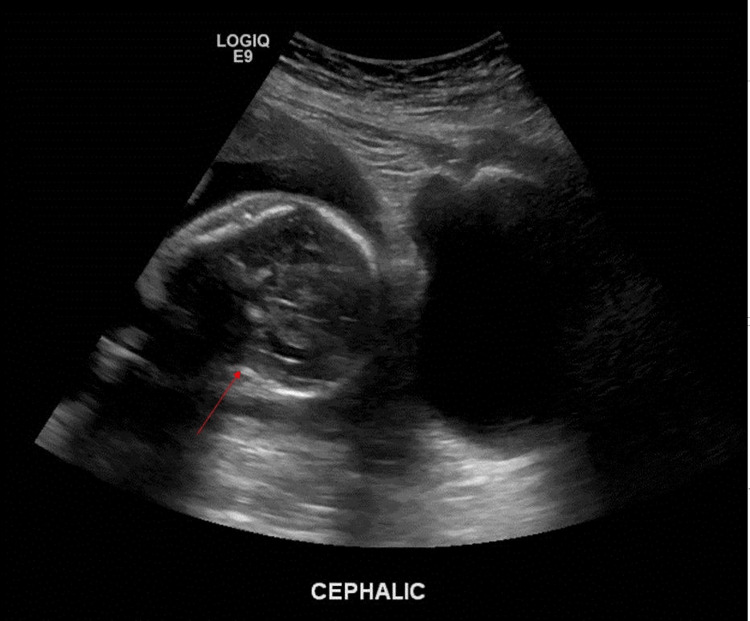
Ultrasound at 24 weeks of gestation shows a single viable uterine pregnancy (red arrow)
